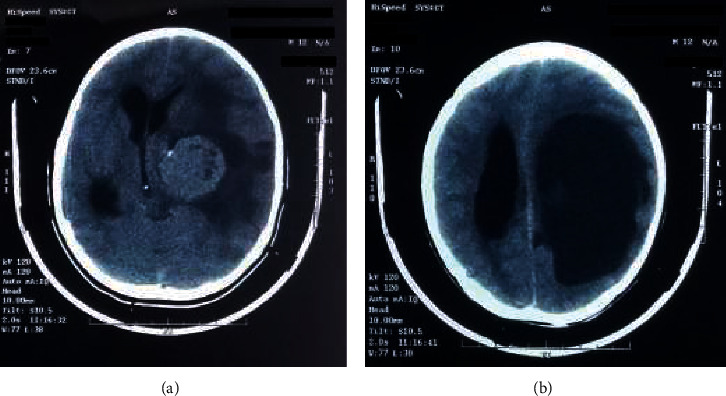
Preoperative simple CT of the brain. (a) 10 × 7 cm mass in the posterior horn of the ventricle. (b) Lesion at the left parietal region accompanied by secondary ventricular dilation.
